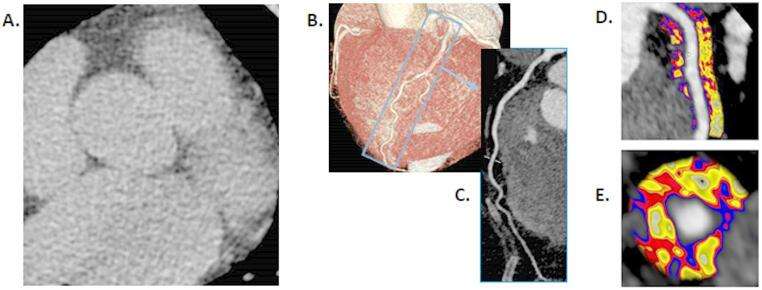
A case example of a 67-year-old female patient with mixed dyslipidaemia. Panel A demonstrates a single axial slice of the baseline coronary calcium score, which is normal with an Agatston score of 0; Panel B demonstrates a curved multiplanar reconstruction of this patient’s CT coronary angiogram; Panel C provides a reconstruction of the left anterior descending artery (LAD) from this CT, which is normal with no evidence of plaque; and Panels D and E demonstrates fat attenuation index (FAI) analysis of the same LAD from panel C, which is significantly abnormal (FAI − 69.4HU and FAI-score in the 87th percentile for age and gender) shown visually by the red and blue colouring in the perivascular space
